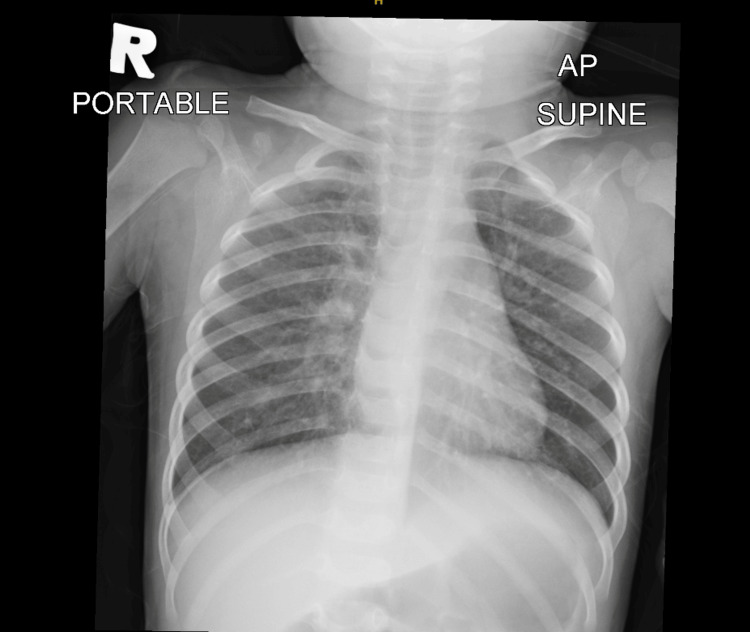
Chest radiograph on admission. Portable anteroposterior (AP) supine chest radiograph of an infant showing hyperinflated lungs with flattened diaphragms and bilateral perihilar interstitial infiltrates, more prominent in the right lower and left perihilar zones. The cardiac silhouette is within normal limits. No focal consolidation, pleural effusion, or pneumothorax is observed. These findings are consistent with viral-induced pneumonitis and airway inflammation secondary to *Bordetella pertussis *infection, with possible contribution from concurrent SARS-CoV-2 infection.
